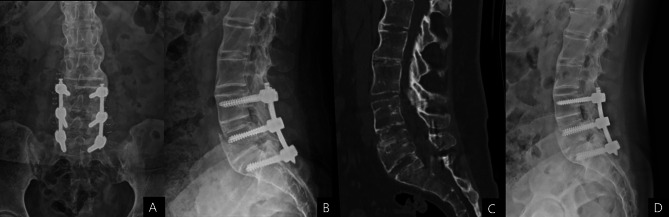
A & B) Simple radiographs show short-segment fixation without fusion by percutaneous technique. C & D) Computed tomography scan and lateral radiograph at 8 months postoperative reveal bony union of L5
